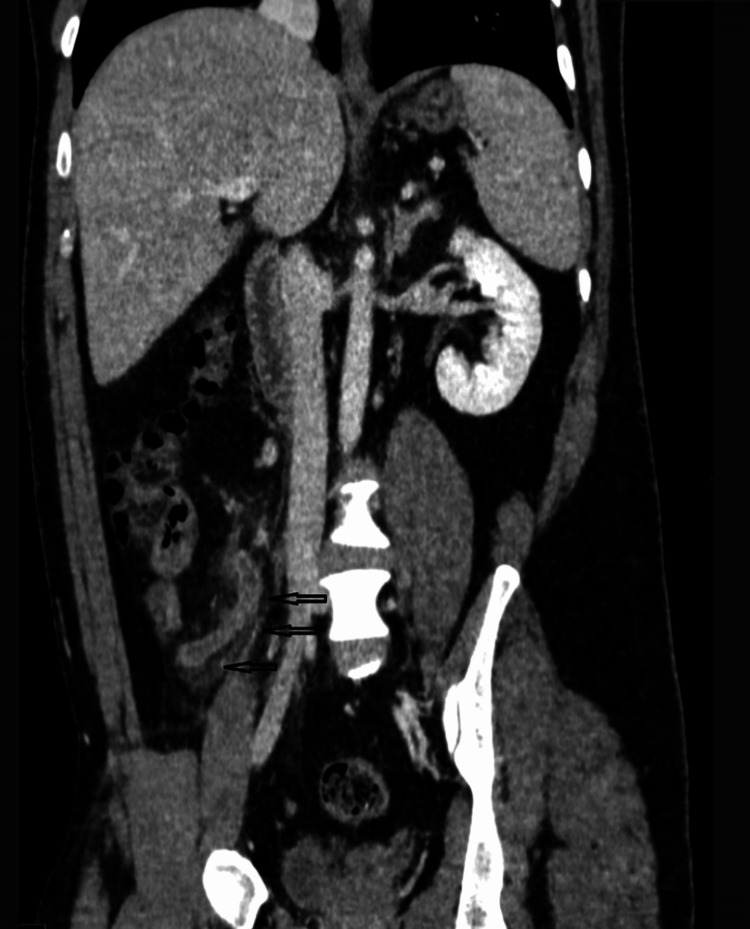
Coronal CT abdomen demonstrating features of acute appendicitis A CT scan of the abdomen in coronal view reveals inflammatory fat stranding surrounding the appendix. The black arrows indicate the appendix, with surrounding stranding consistent with acute appendicitis.
